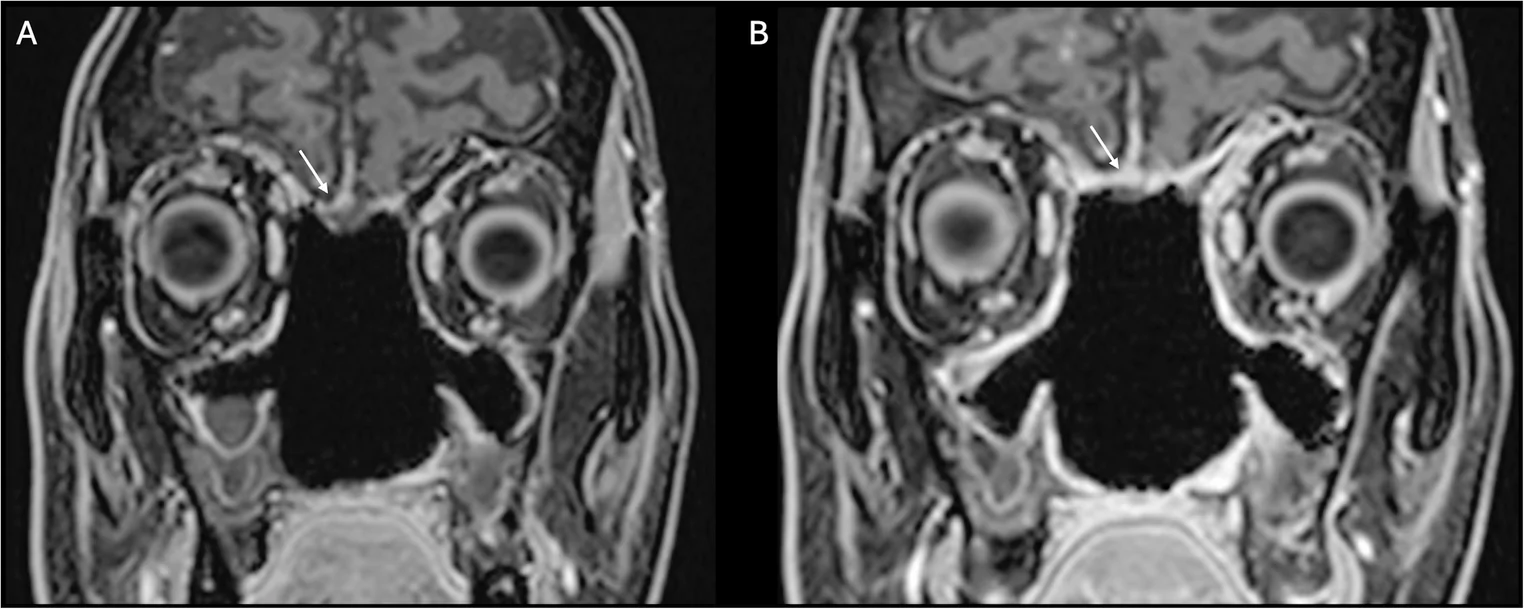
Contrast-enhanced coronal T1-weighted MRI images showing the radiological evolution following dural reconstruction with autologous material and application of a septal flip-flap after endoscopic endonasal right dural resection for an intestinal-type adenocarcinoma. In contrast to the use of xenogenic materials, both in the immediate postoperative period (A, 2 months postoperatively) and in the medium term (B, 8 months), the absence of intracranial contrast enhancement is evident, with the characteristic contrast uptake at the site of the pedicled flip-flap (indicated by the white arrow) which, in this case, is more pronounced at 8 months
